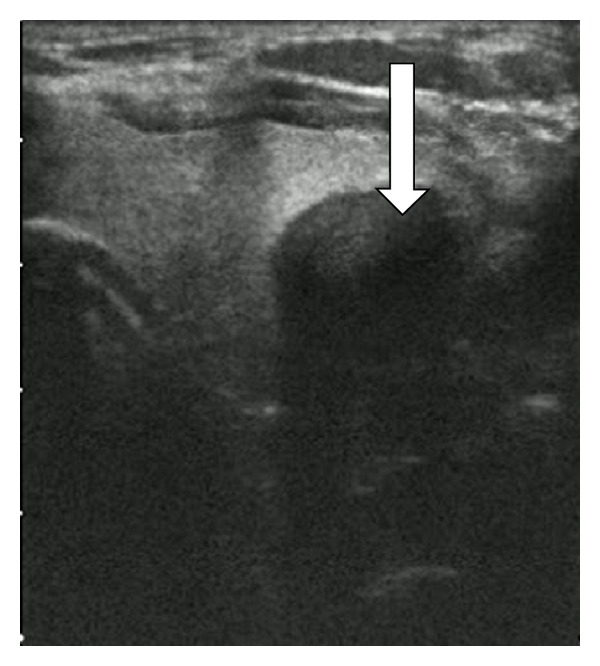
Ultrasonography showing a heterogeneously hypoechoic giant mass with undetermined inferior margins.
